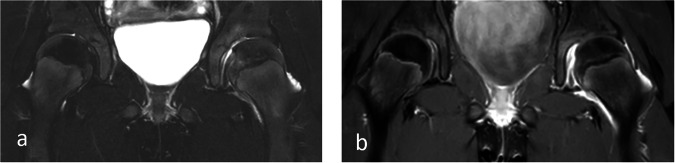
Nine-year-old girl with psoriasis-related JIA and pain in her left thigh. MRI, with (a) a coronal T2-weighted SPAIR sequence, showed a sliver of joint fluid in her left hip, whilst the T1-weighted, fat-saturated post-contrast image (b) showed vivid synovial enhancement, consistent with arthritis, underscoring the need for intravenous contrast administration to assess active inflammation
